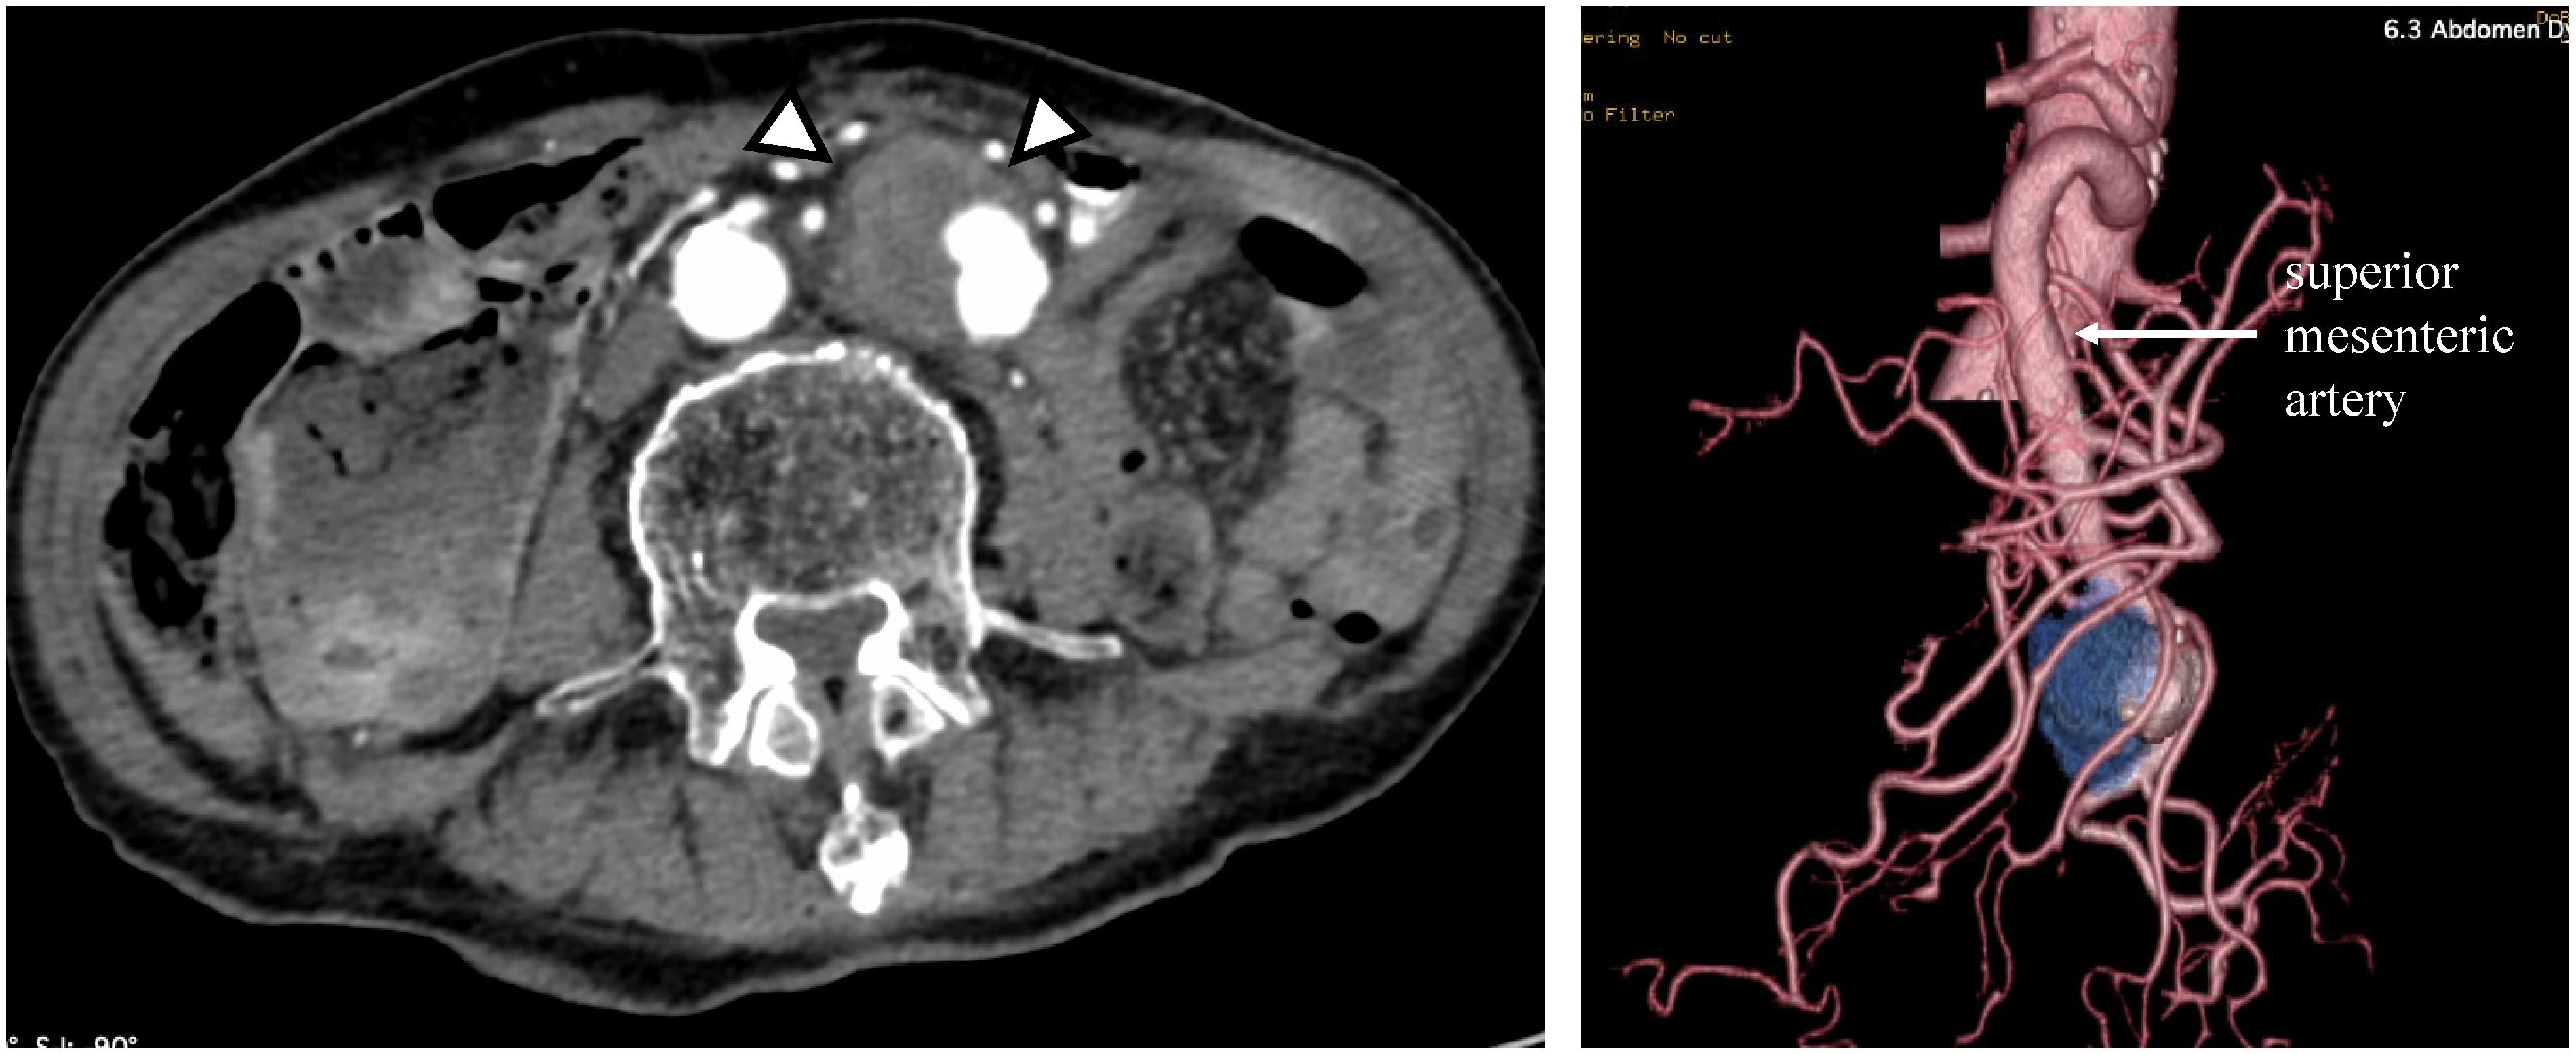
Fig. 1 Contrast-enhanced computed tomography findings. A 36-mm superior mesenteric artery aneurysm (arrow head) was located 11 cm distal to the origin of superior mesenteric artery and contained an intramural thrombus.
